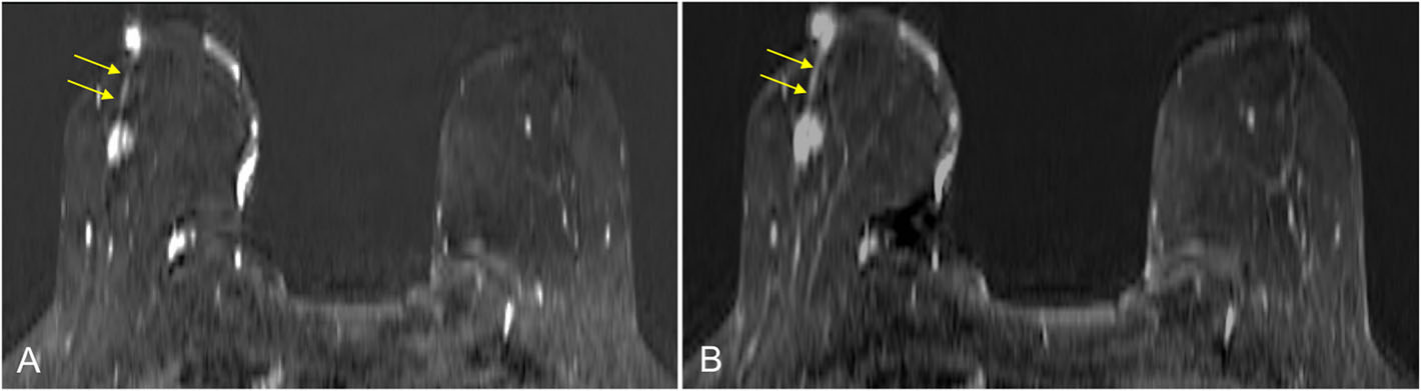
Pathological enhancement in the nipple-areolar complex. A 71-year-old woman. Axial contrast-enhanced T1-weighted spoiled gradient-echo (subtracted) images show asymmetric irregular nodular early enhancement (a) that is maintained in late phases (b) secondary to involvement by invasive ductal carcinoma. Irregular-shaped masslike enhancement in the middle third of the junction of the outer quadrants in the right breast with linear uptake and segmental distribution to the nipple-areolar complex, compatible with an intraductal component (arrows)
